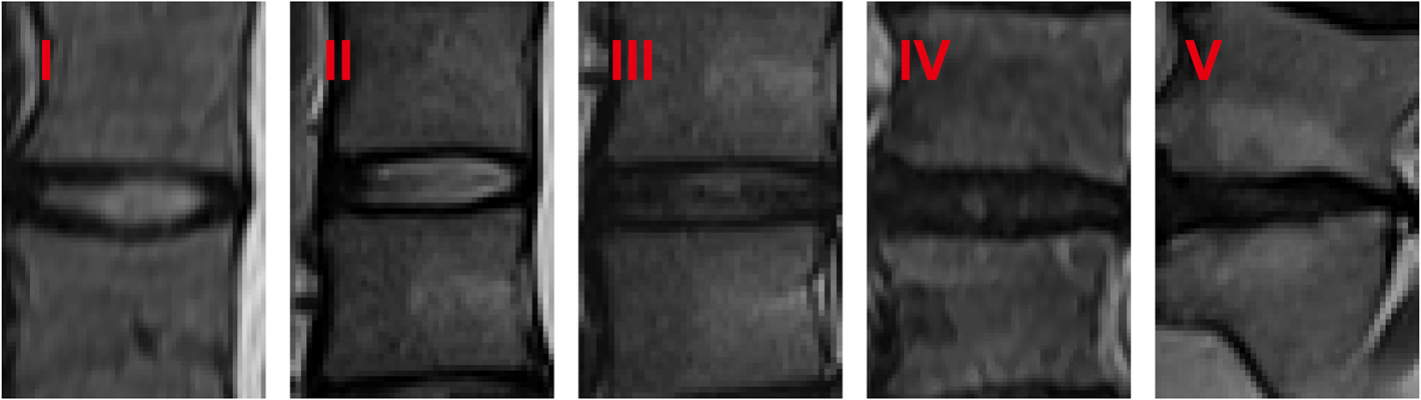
The classification of Pfirrmann grade. On T2-weighted image, grade I—the structure of the disk and disk height are normal, with a bright white signal intensity. Grade II—the structure of the disk is abnormal and the disk height is normal, with a white signal and a clear distinction between nucleus and anulus. Grade III—the structure of the disk is abnormal and the disk height is normal or slightly decreased, with an average gray signal intensity and an unclear distinction between nucleus and anulus. Grade IV—the structure of the disk is abnormal and the disk height is normal or moderately decreased, with a dark gray signal intensity and a lost distinction between nucleus and anulus. Grade V—the structure of the disk is abnormal and the disk space is collapsed. The distinction between nucleus and anulus is lost
